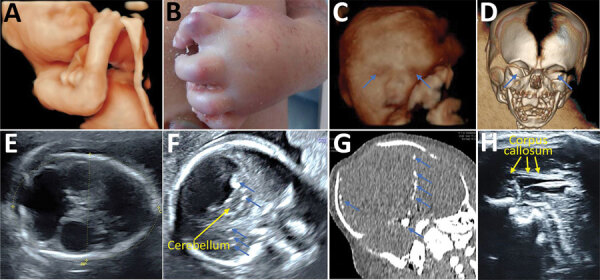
Prenatal ultrasound, computed tomography, and postmortem aspect of a fetus with congenital Zika syndrome related to maternal prolonged viremia in patient (case no. 122) in a cohort study of pregnant women admitted to Centre Hospitalier de l’Ouest Guyanais, French Guiana, January 1–July 15, 2016. The mother had symptomatic acute Zika virus infection at 8 weeks’ gestation (and had ongoing viremia until birth of her stillborn child with signs of congenital Zika syndrome. Severe microcephaly, ventriculomegaly, and calcifications were detected by ultrasound at 13 weeks’ gestation. Overall, this fetus had arthrogryposis detected on 3-D ultrasound (A) and postmortem (B); severe bilateral microphthalmia (blue arrows) detected on 3-D ultrasound (C) and fetal computed tomography (D); microcephaly with atrophic cortex detected on ultrasound (E) showing a head circumference of 160 mm at 25 weeks’ gestation (−5 SDs); ventriculomegaly detected on ultrasound (E); brain calcifications (blue arrows) detected on ultrasound (F) and computed tomography (G); pontocerebellar hypoplasia (yellow arrows) detected on ultrasound (F); and corpus callosum dysgenesis (yellow arrows) detected on ultrasound (H).
